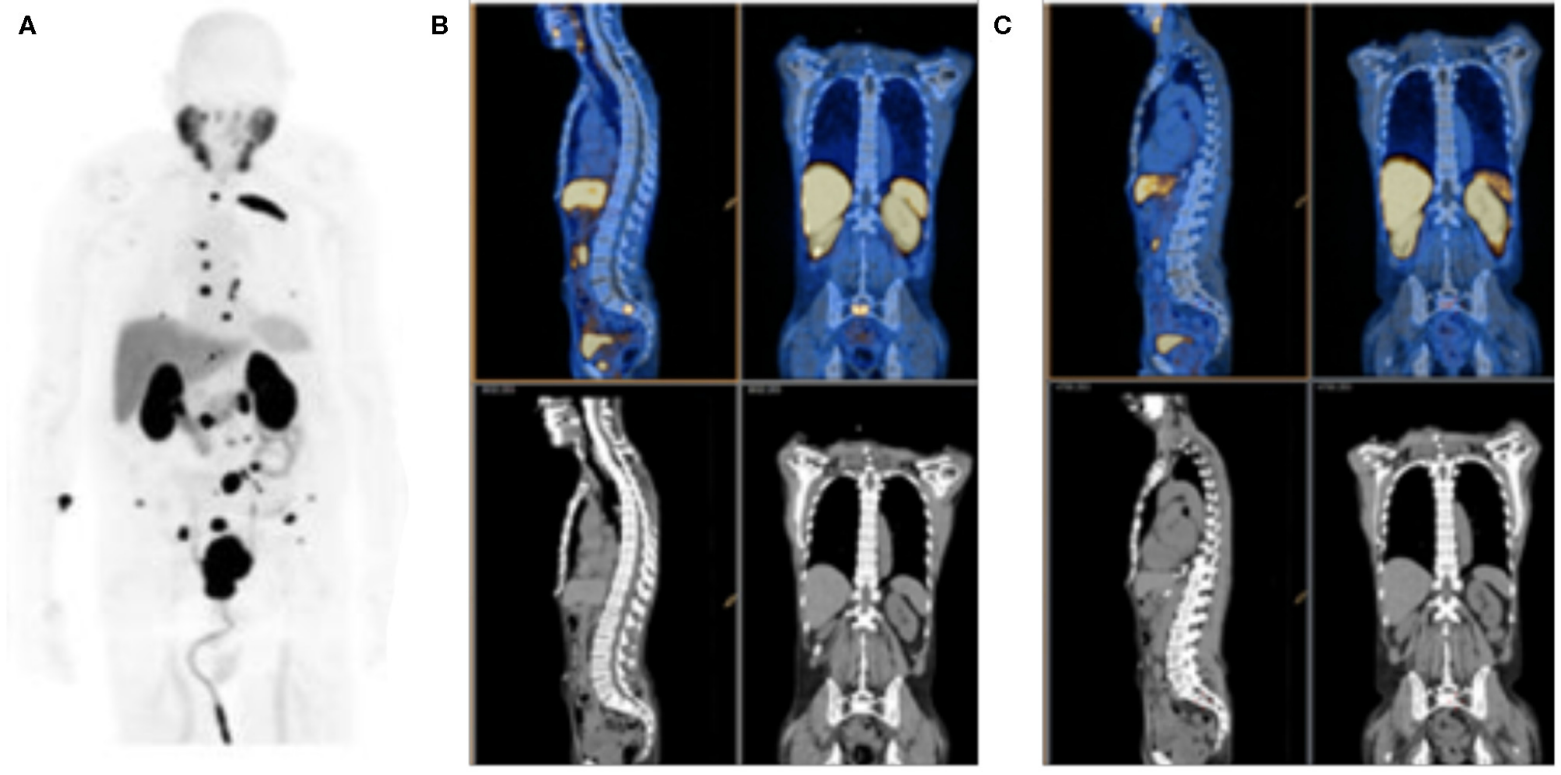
PSMA PET Imaging. (A) Detection of prostate cancer lesions with [^18^F]DCFPyL, along with uptake in kidneys, salivary gland and liver. (B) Pre-treatment [^68^Ga]PSMA-11 and (C) post-treatment PET/CT, top, and CT, bottom, scans obtained on day 9 after initiating combined anti-androgen hormone therapy. Reproduced from (A)(40) and (B,C) (41).
